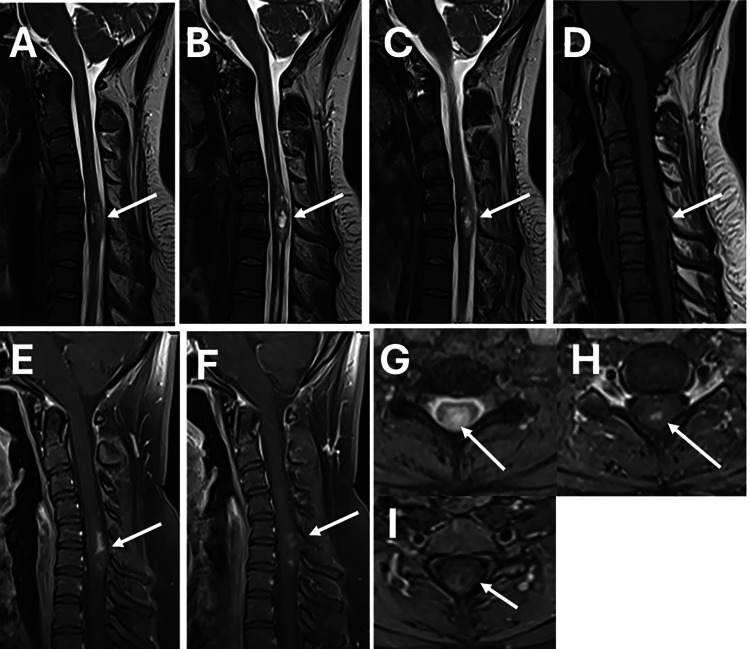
Preoperative magnetic resonance images of the patient (A) The lesion enlarging the spinal cord at the C5-6 level should be seen on T2-weighted MRI (white arrow) (B) On another T2-weighted MR slice at the same level, the lesion is heterogeneous (iso and hyperintense) (white arrow) (C) On another T2-weighted MR slice at the same level (white arrow) (D) On T1-weighted MR image, the center of the mass is hypointense and the periphery is isointense (white arrow) (E,F) T1 contrast-enhanced MR images show that the mass is irregularly circumscribed and heterogeneously contrasted (white arrow) (G) T2 axial MR image shows that the lesion center is hyperintense and intramedullary (white arrow) (H,I) T1 axial contrast-enhanced MR images show heterogeneous contrast enhancement and intramedullary localization of the spinal cord (white arrow)
